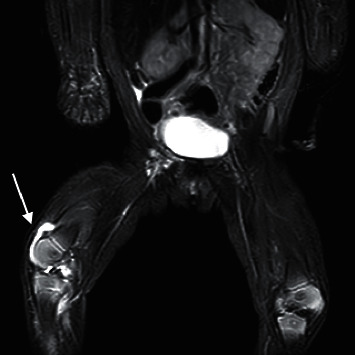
MRI of the patient's right knee showing small to moderate right knee effusion compared to a normal left knee. There is no myositis or soft tissue fluid changes.
